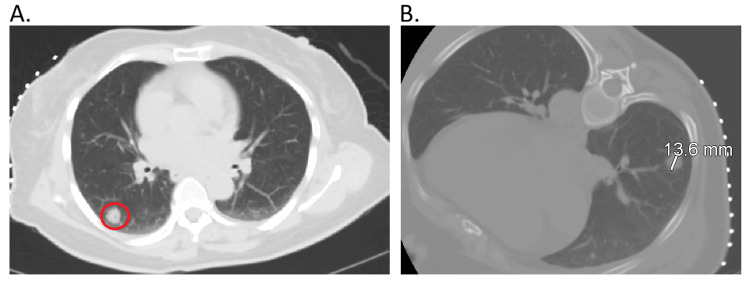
Computed tomography of the chest, abdomen, and pelvis with contrast A. The red circle indicates a subsolid nodule in the right lower pulmonary lobe. B. The same nodule is noted to be 13.6 mm in diameter.
